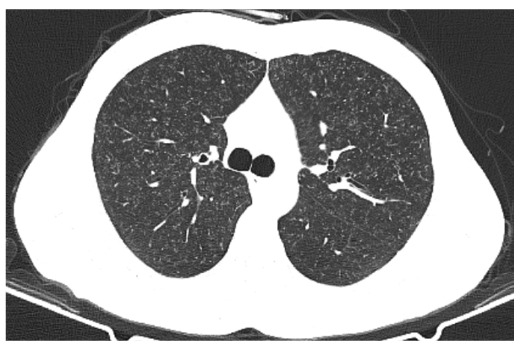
Axial noncontrast thoracic computed tomography image showing multiple micronodules randomly distributed in the bilateral lungs (miliary nodular pattern).
